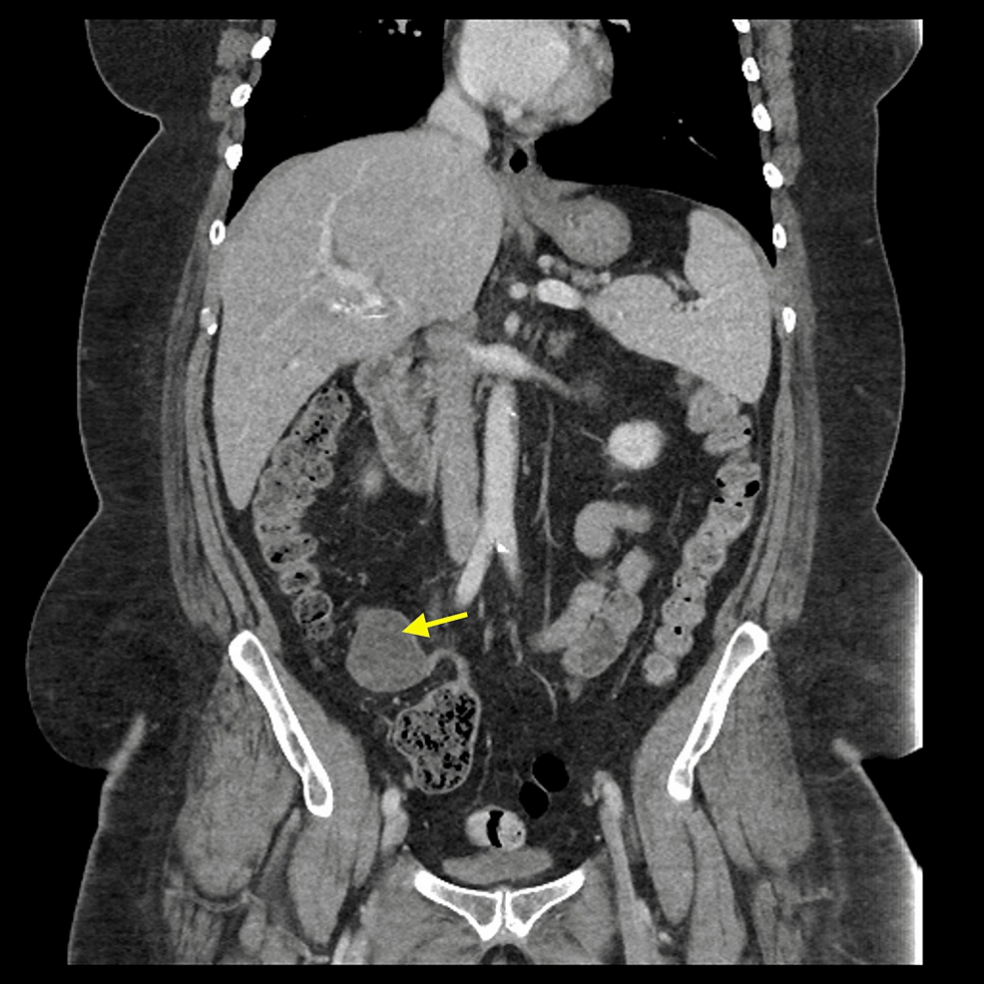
CT abdomen and pelvis with contrast (Case 5). Coronal view showing a cystic mass in the distal appendix. Pathological examination of the mass showed LAMN. LAMN, low-grade appendiceal mucinous neoplasm
